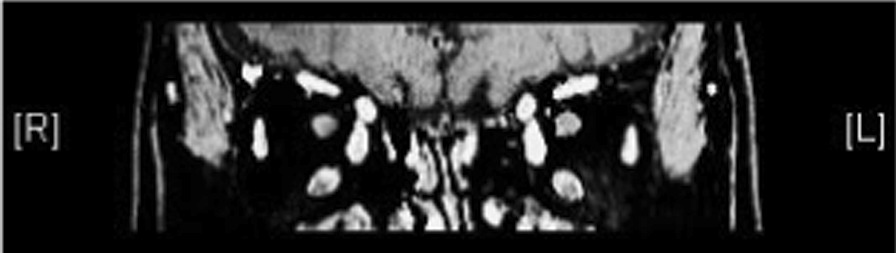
Gadolinium-enhanced T1-weighted MRI image of bilateral orbicularis oculi muscle, coronal view. Showing no abnormal mass lesions or enhancement
